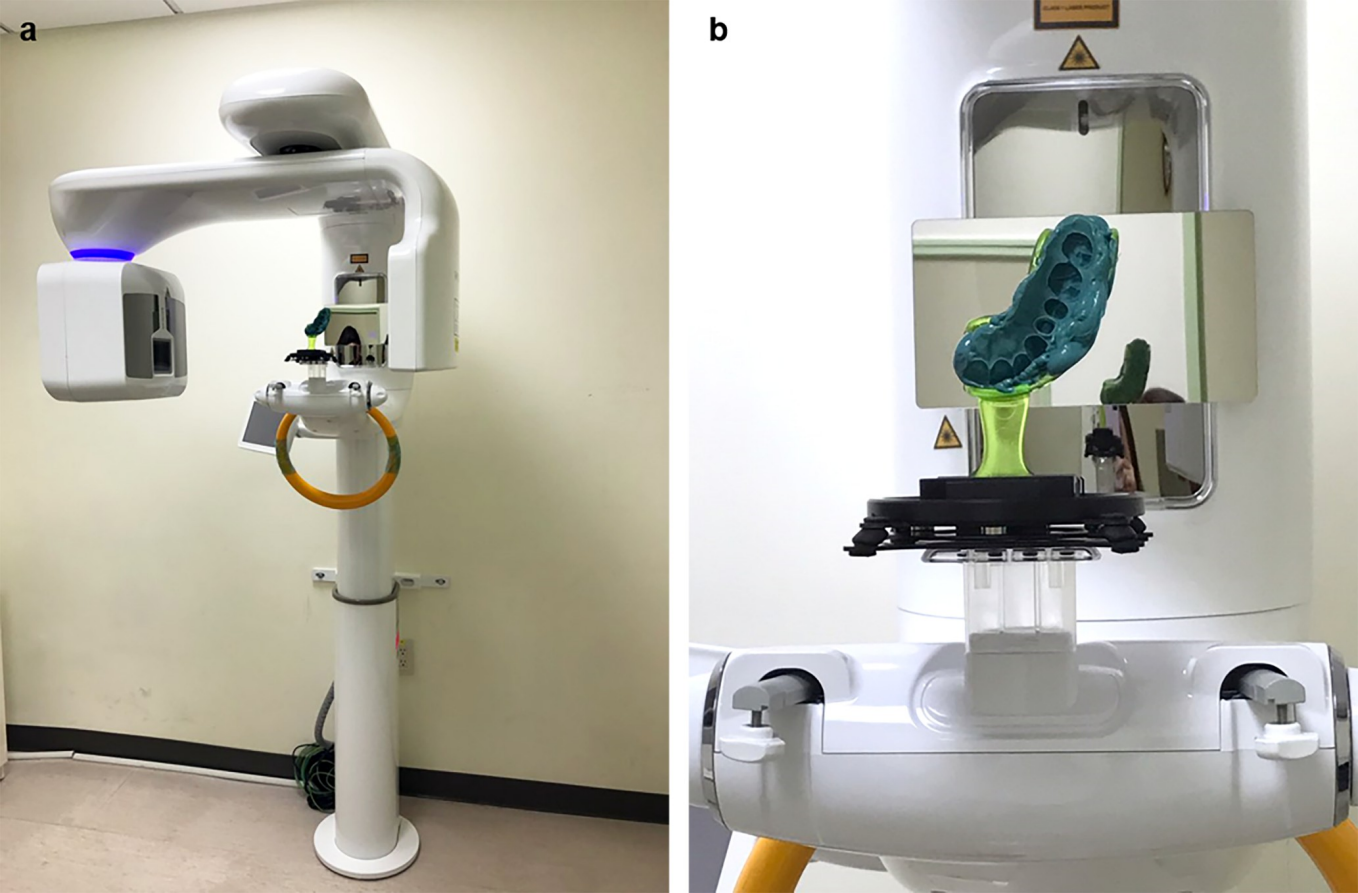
Cone-beam computed tomography (CBCT) scanning. (a) CBCT apparatus (RAYSCAN α+) and (b) a polyvinylsiloxane impression fixed on a customized chin rest provided by the manufacturer for scanning.
